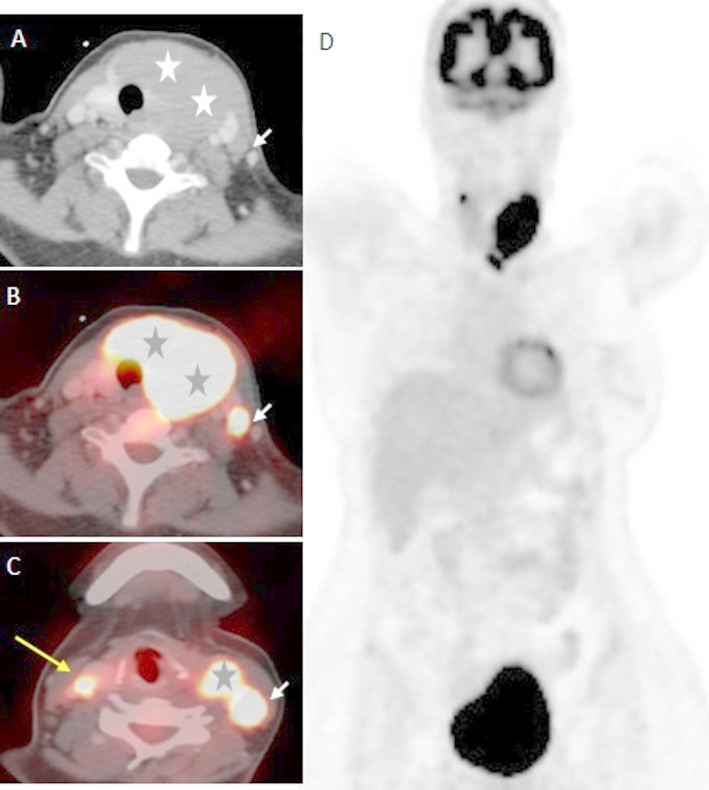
Baseline PET-CT of a patient PTL Axial post-contrast CT (A) and axial fused PET-CT (B&C) revealed metabolically avid diffuse left thyroid and isthmic lesion (stars) displacing left CCA and internal jugular vein laterally without vascular invasion (SUVmax 25). Small right thyroid lobe nodule with similar metabolic activity (long arrow). Few left level III cervical LNs, the largest of SUV max about 21 (short arrows). Coronal reformatted maximum intensity projection (MIP) image (D) confirms the absence of other metabolically active lesions
